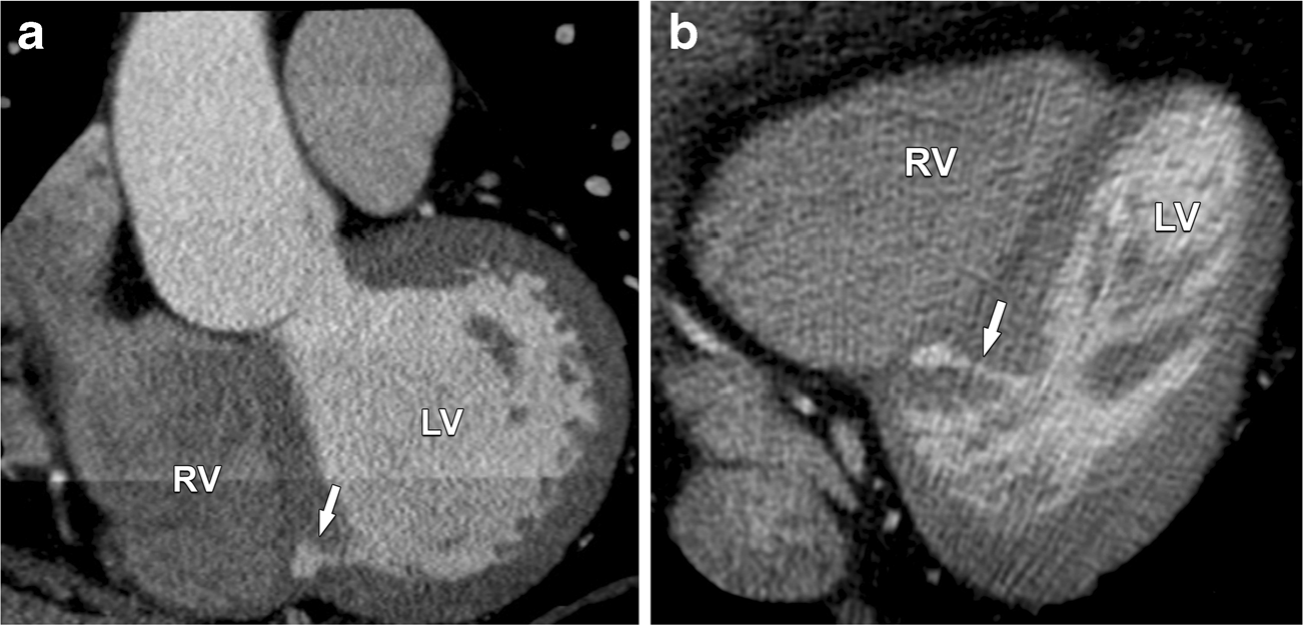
Coronal oblique (a) and axial (b) contrast-enhanced CT nicely show a ventricular septum diverticulum (*arrow*). There is a defect originating from the left ventricle (LV) extending in the muscular interventricular septum without reaching the right ventricle (RV). This entity has no hemodynamic importance but can increase the risk of endocarditis
